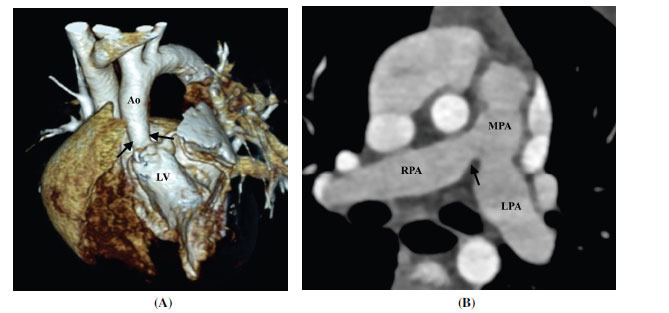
Supravalvular aortic stenosis in a case of William syndrome. (A) Volume rendered technique image showing supravalvular aortic stenosis (arrow) (B) Axial contrast-enhanced computed tomographic angiography image showing mild focal stenosis at the ostium of the right pulmonary artery (arrow).
